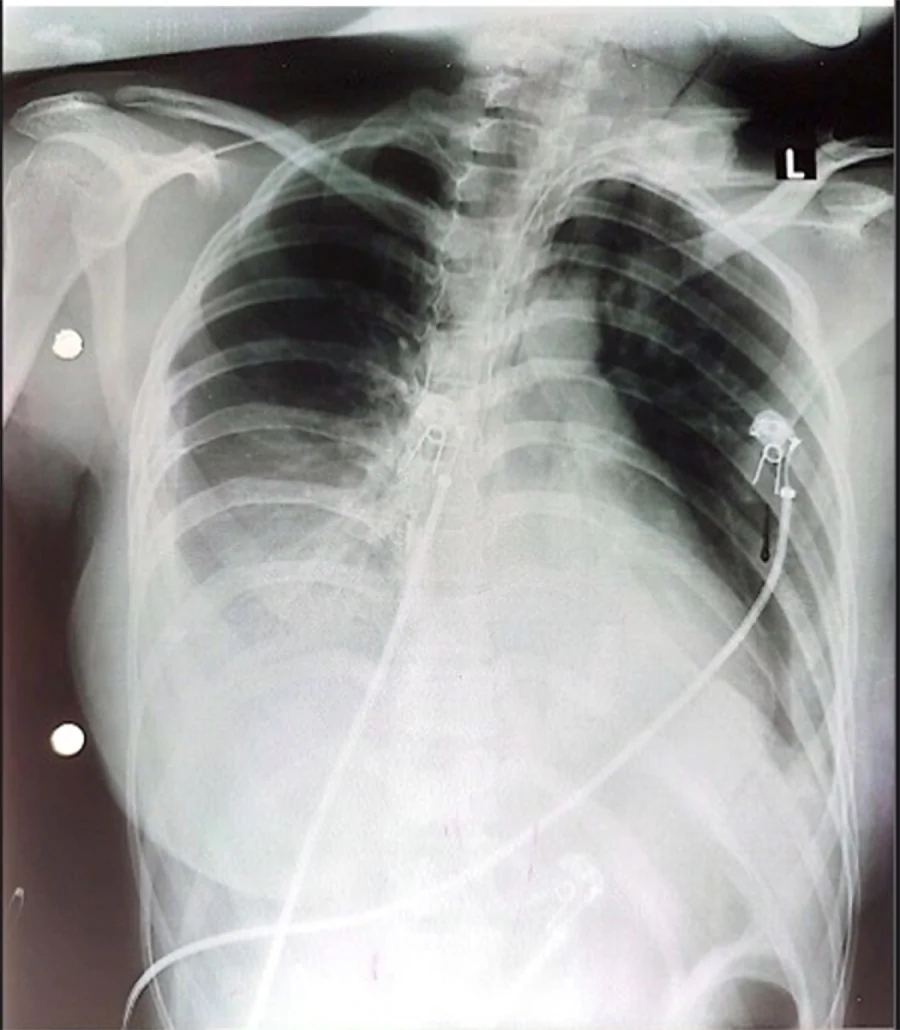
Chest radiograph showing right-sided pleural effusion.
